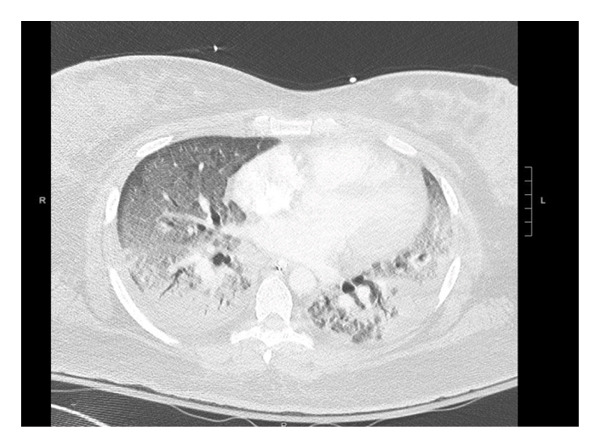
CTA chest showing diffuse, patchy infiltrates throughout both lungs.
